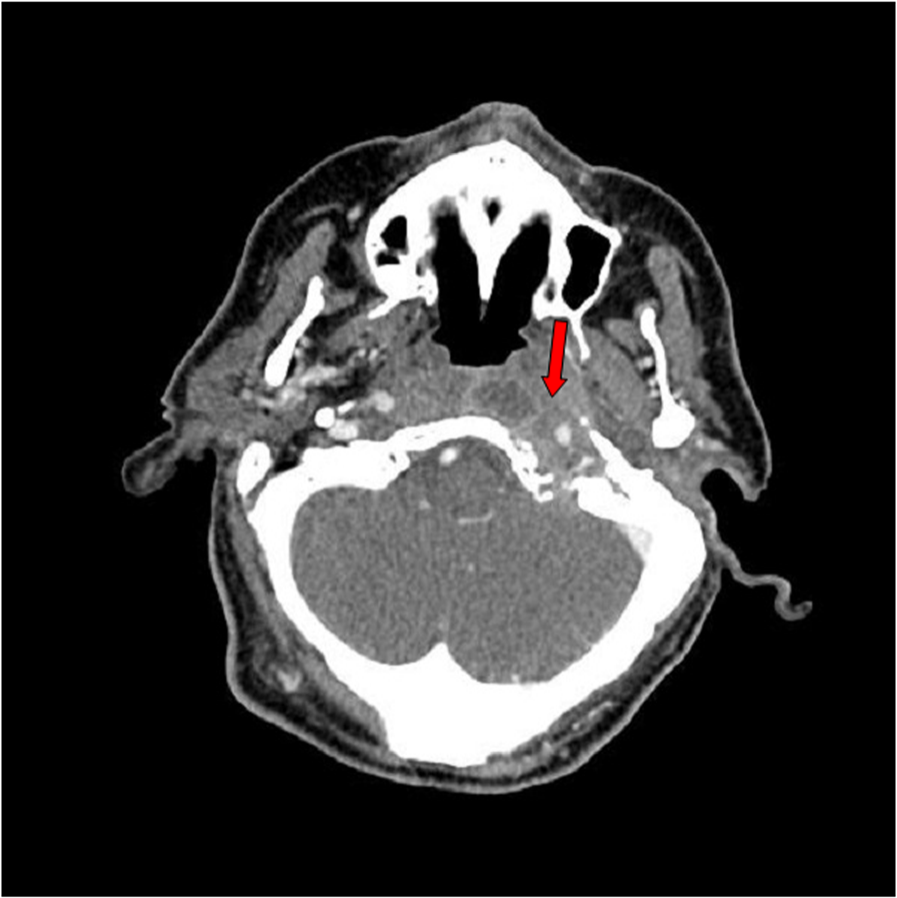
Cranial computed tomography scan shows a mass of soft tissue (*arrow*) surrounding the internal jugular vein and the carotid artery at the left jugular foramen with signs of bone erosion and destruction. The lesion extends medially and causes bone destruction of the left occipital condyle and the left side edge of the clivus and erosion of the posterior edge of the oval hole. In the petrous bone it extends to the middle ear and causes erosion of the anterior wall of the tympanic cavity. The mass goes along the petrous carotid reducing its caliber and causing bone destruction of the anterior edge of the carotid canal extending to the petrous apex
